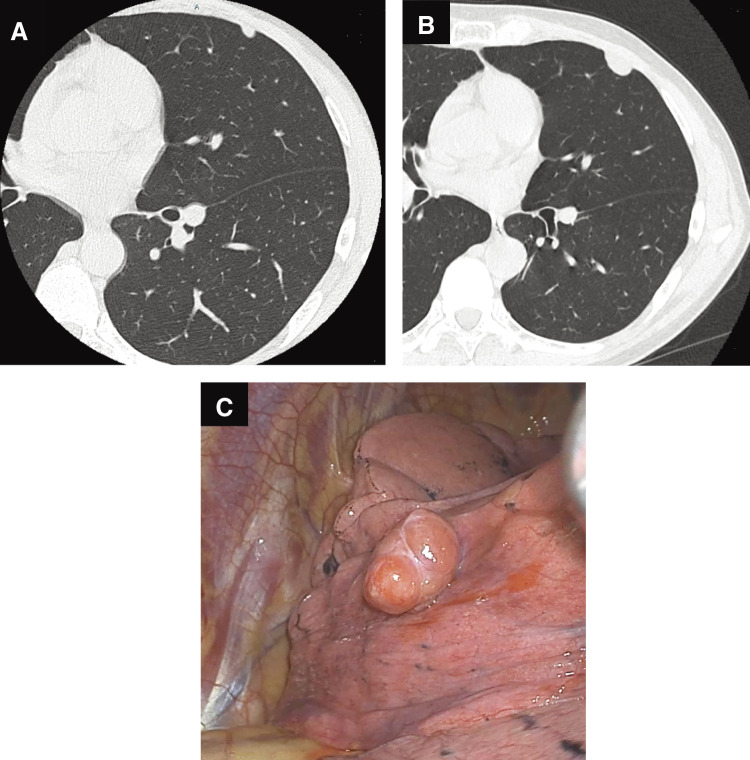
(A–C) CT images and intraoperative appearance of the lesion. (A and B) CT images showed that the tumor had clearly increased in size over the past 10 years. (C) The tumor was grossly protruding from the peripheral lung parenchyma with no implication of invasion into the surrounding tissue. CT, computed tomography
